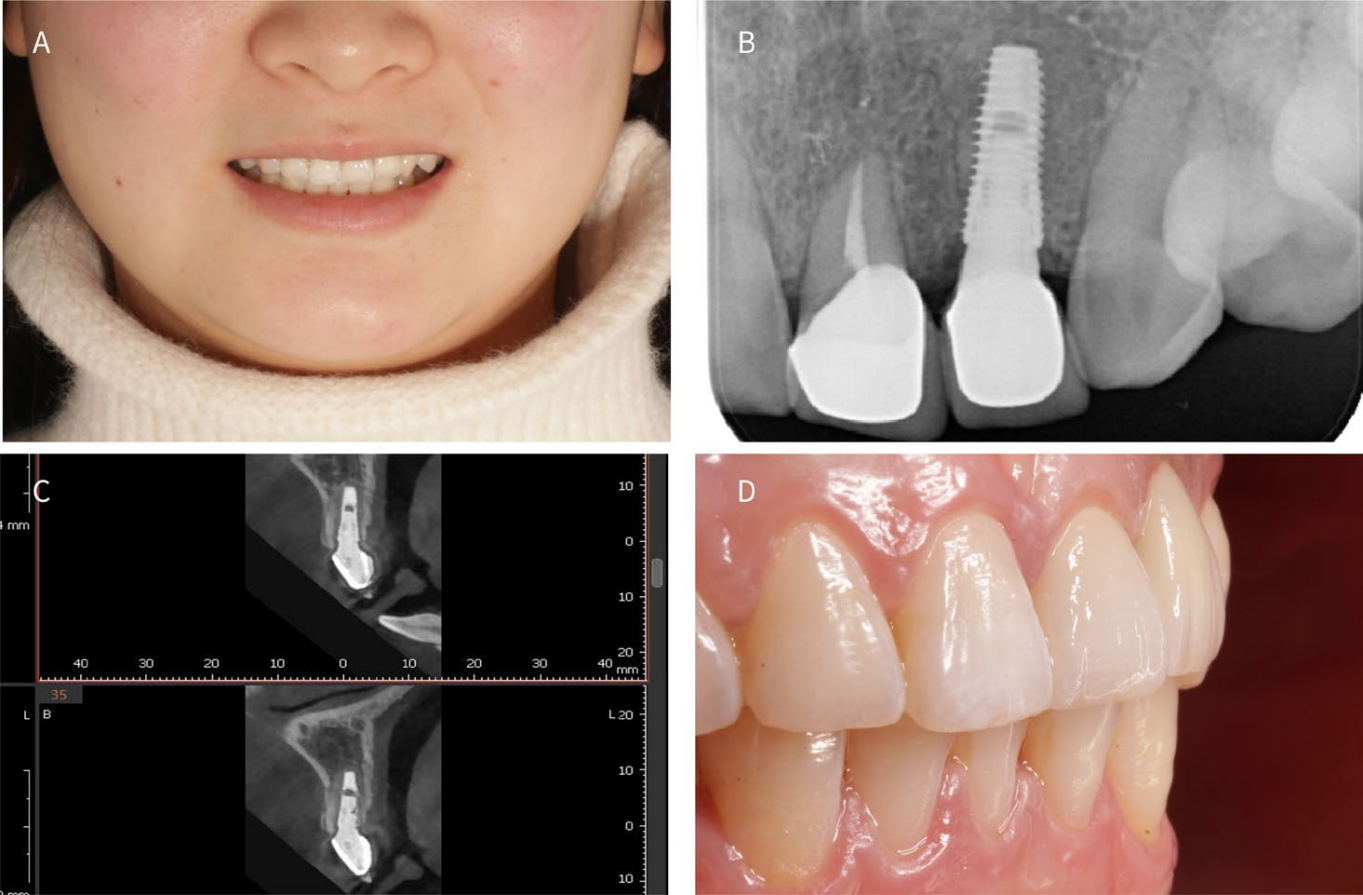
(A) Frontal smile view after definitive restoration placement; (B, C) Post‐restoration radiographic images, no hard‐tissue resorption was detected; (D) Final restoration intraoral views.
